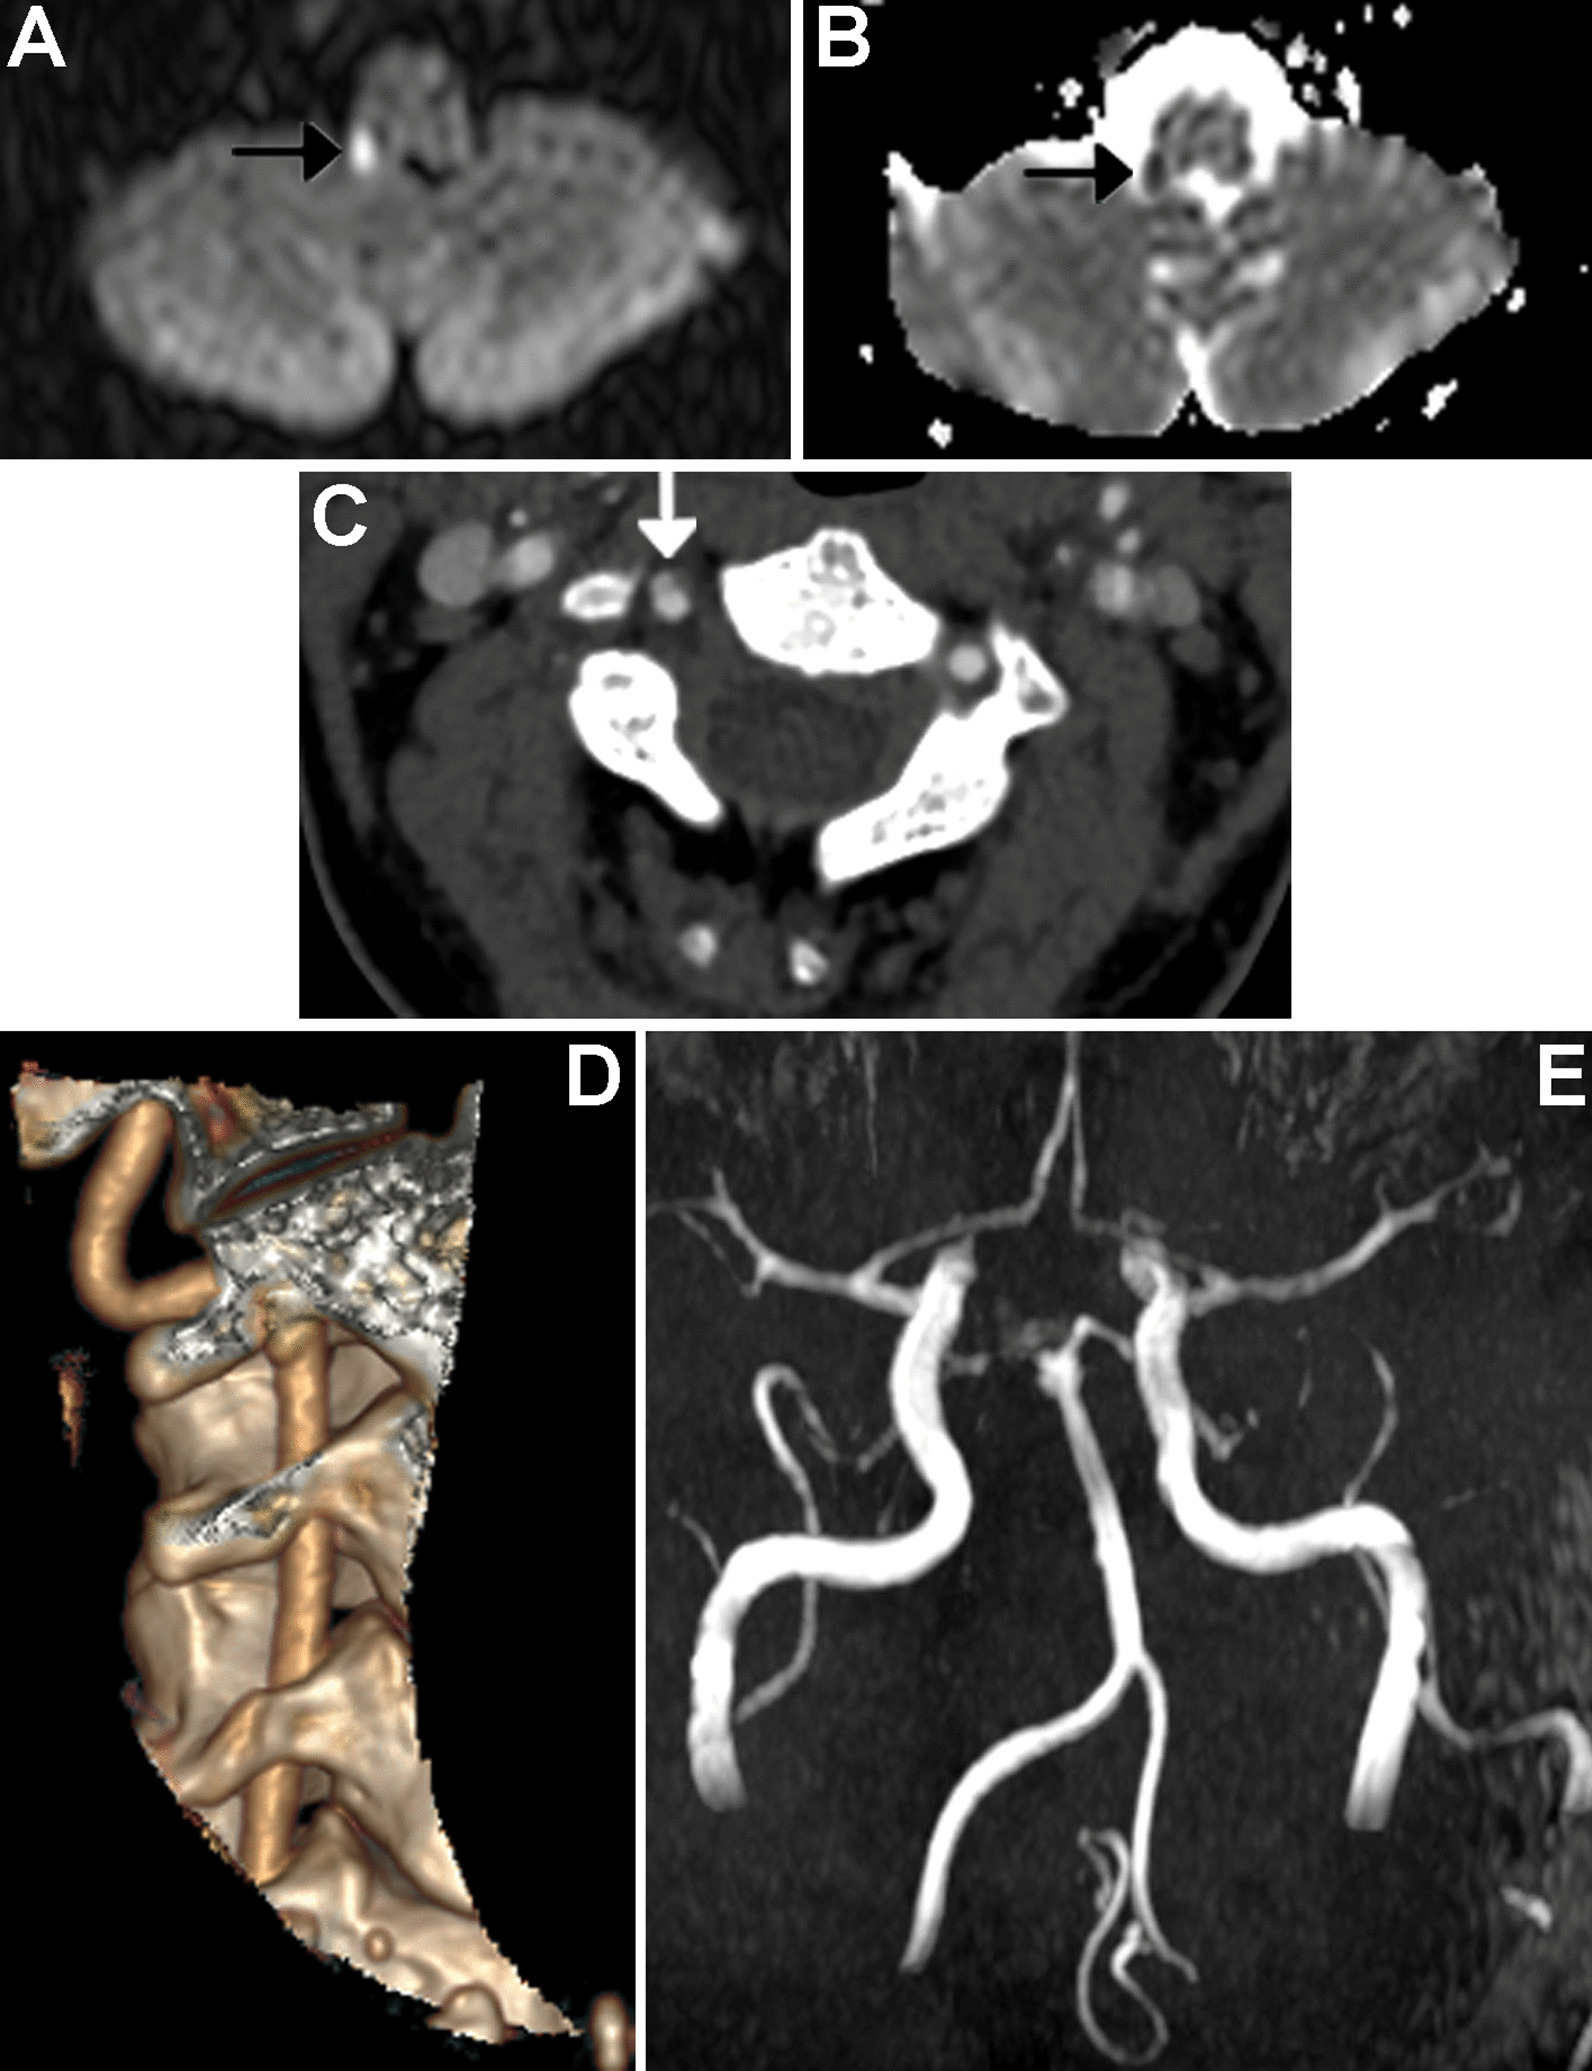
Right vertebral artery dissection with ischemia in the posterolateral medulla oblongata. In DWI (a) and ADC map (b) the arrow shows a punctate, shiny ischemic lesion, with typical reduction of ADC in the right posterolateral medulla oblongata. c and d CT angiography (axial and 3D reformat, c and d, respectively) showing a focal dissection of the V2 distal segment of the right vertebral artery, with the arrow in figure c pointing to the dissection. e MRI angiography (time of flight, TOF) showing the absence of visualization of right PICA
